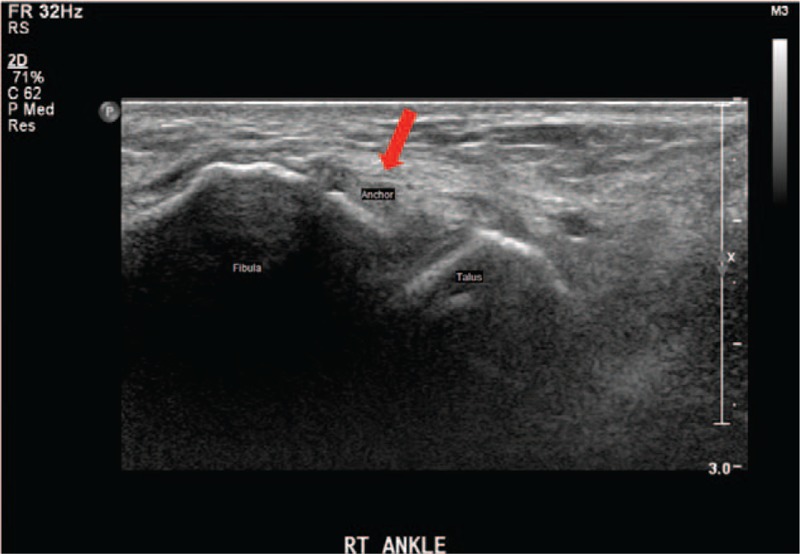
Preoperative ankle ultrasonography shows the suture anchor protruding posteriorly at the fibula tip.
